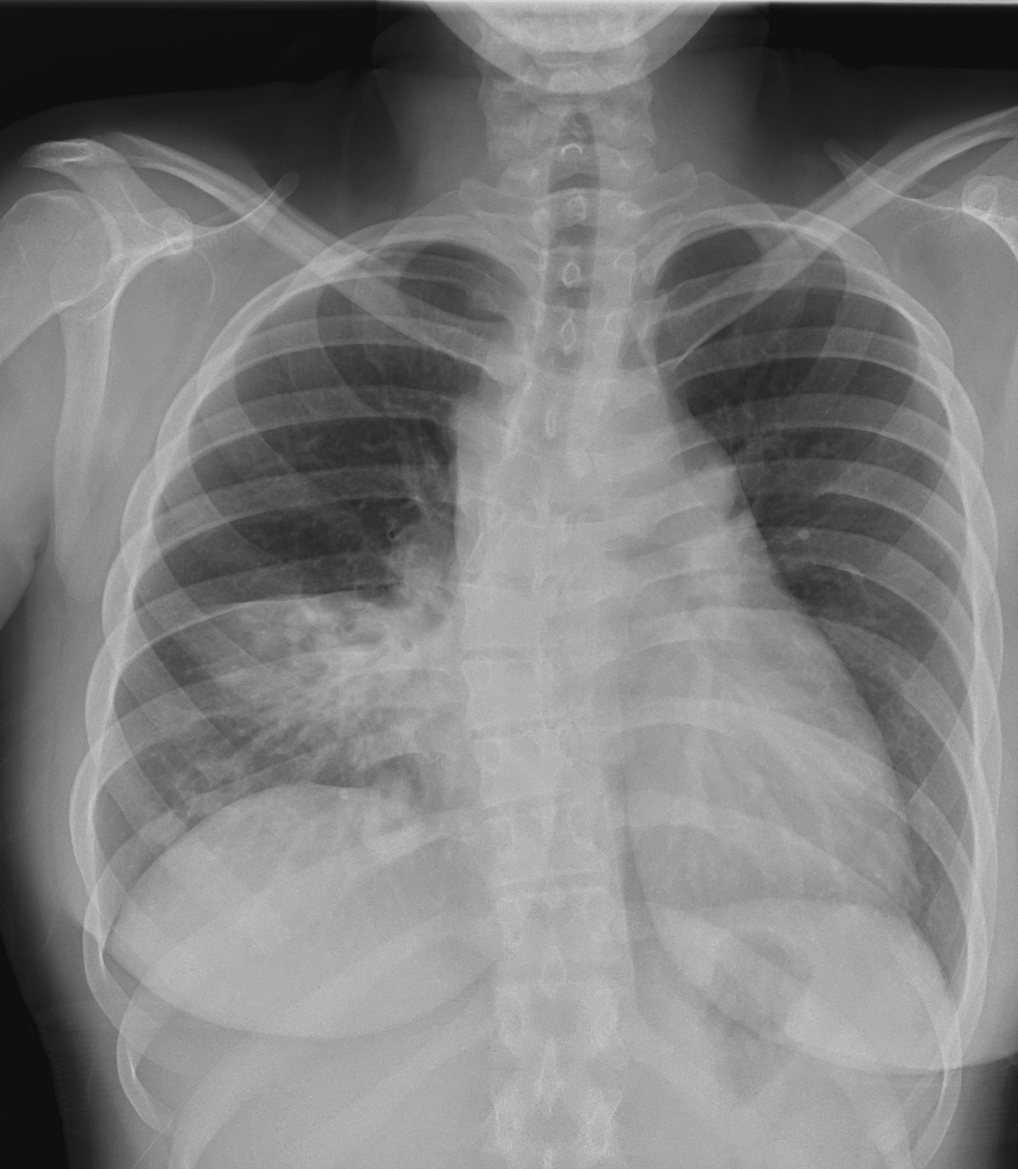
Chest X-ray: anteroposterior erect view Mediastinal widening and consolidation in the right middle lobe, as well as air bronchograms bilaterally.
